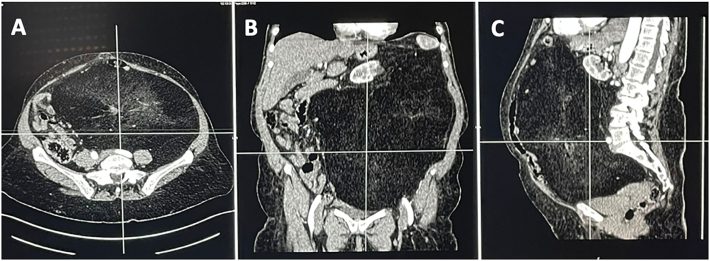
A, B, C. Abdominal contrast-enhanced computed tomography (CECT) showing a heterogeneous and hypodense (fat density) giant retroperitoneal mass, measuring 36 × 32 × 28 cm, compressing and displacing the surrounding intra- and retro-peritoneal organs and vessels (A axial view, B coronal view, C sagittal view).
